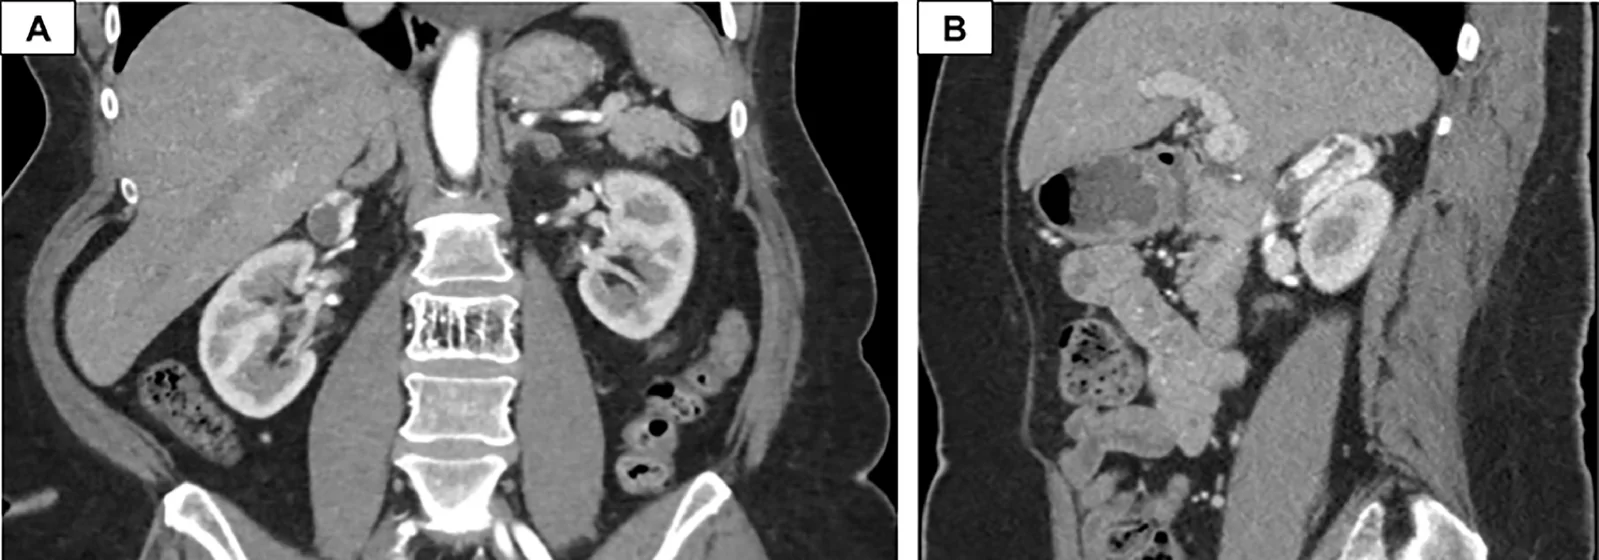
(A) Coronal arterial-phase CT demonstrates the enhancing right perirenal mass closely opposed to the right kidney and adrenal region. (B) Sagittal arterial-phase CT demonstrates a thin intervening fat plane between the lesion and the renal parenchyma, confirming an extrarenal origin.
